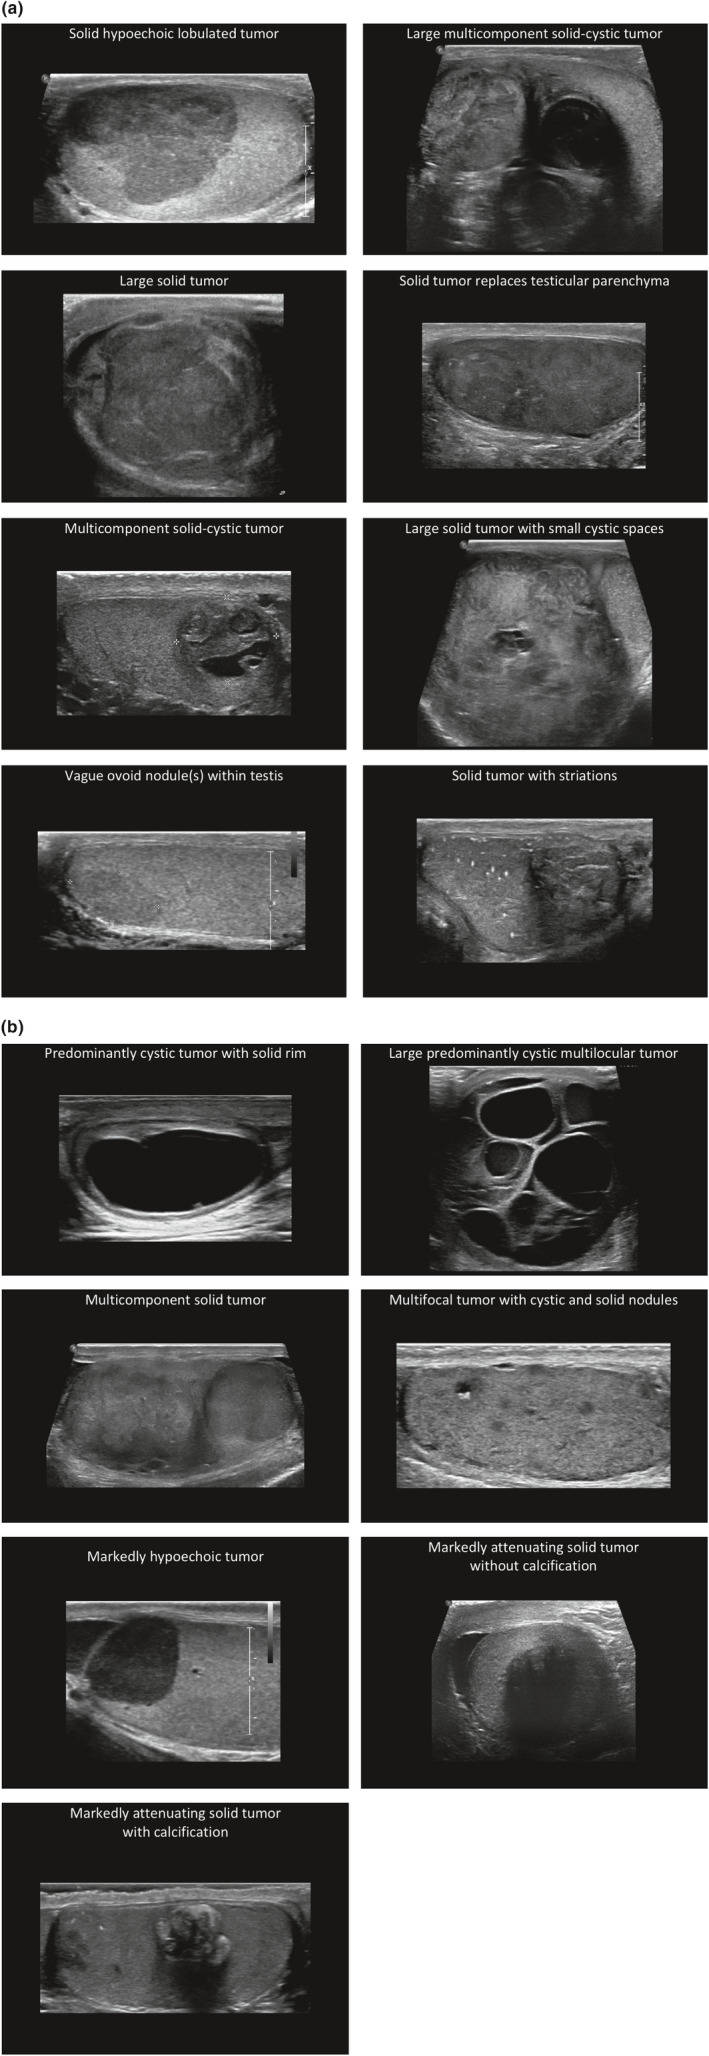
Morphological patterns of testicular tumours as seen on ultrasound.
